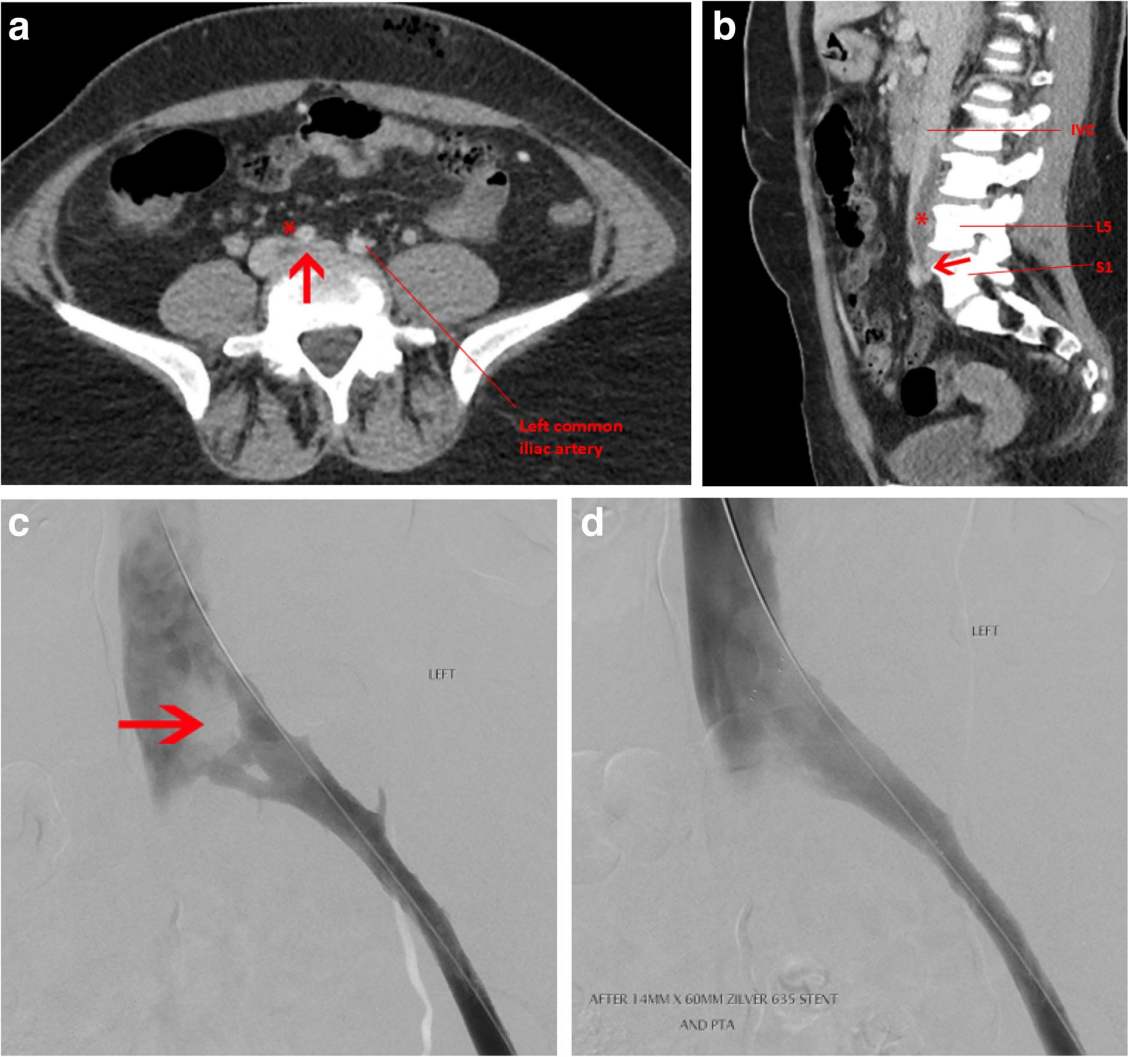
a Anatomy seen in May-Thurner Syndrome: Axial CT venogram of the pelvis shows compression of the left common iliac vein (arrow) between the right common iliac artery (asterisk) and the intervertebral disc of L5-S1. b Anatomy seen in May Thurner Syndrome: Sagittal CT venogram of the pelvis shows compression of the left common iliac vein (arrow) between the right common iliac artery and the intervertebral disc of L5-S1, with acute thrombus in the lower IVC (asterisk). c Frontal iliocaval angiography shows extrinsic compression of the left common iliac vein (arrow). This anatomy is seen in patients with May-Thurner Syndrome. d After placement of self-expanding 14 mm venous stent, improvement in flow is seen as well as resolution of extrinsic compression on the left common iliac vein
